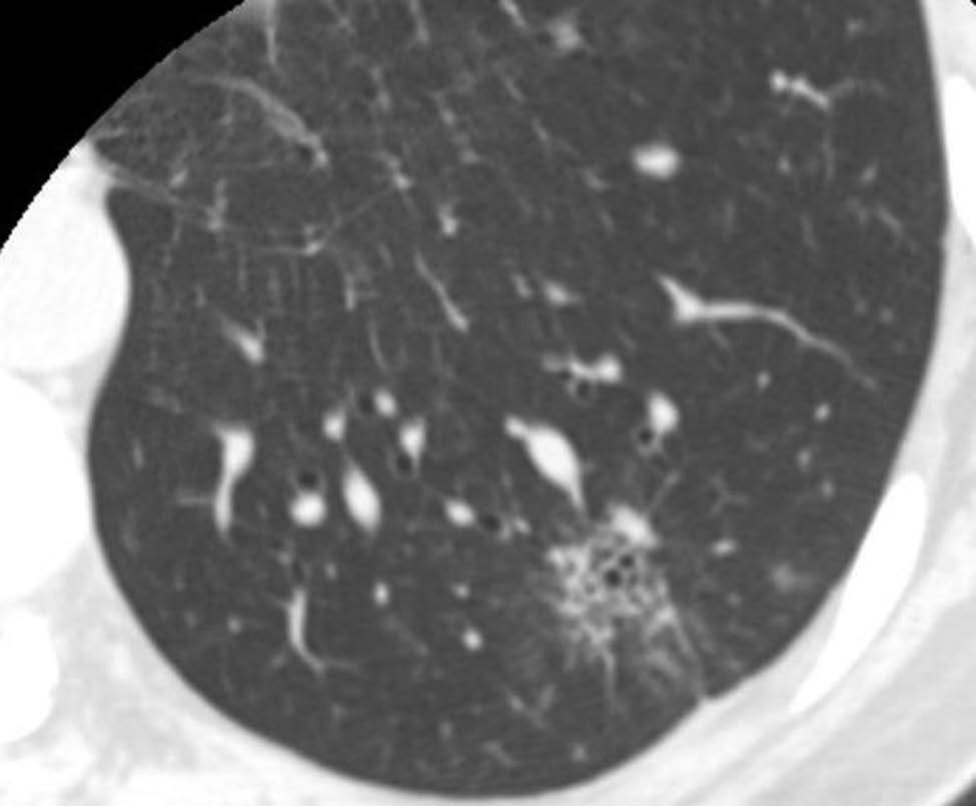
Female, aged 49 years old. Chest CT showed: mixed ground glass nodules in the outer basal segment of the lower lobe of the left lung, with the maximum section diameter of about 14 mm * 14 mm, irregular morphology, unclear boundary, marginal lobation, uneven lesion density, tortuous blood vessels and inflatable bronchi, adjacent pleural adhesion.
